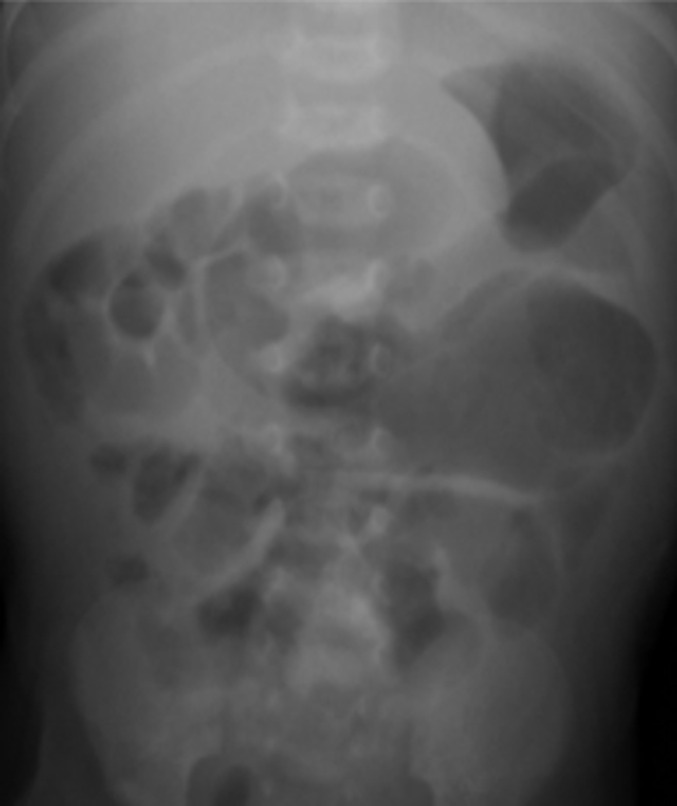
plane abdominal X-ray with a nonspecific dilatation of bowel loops
